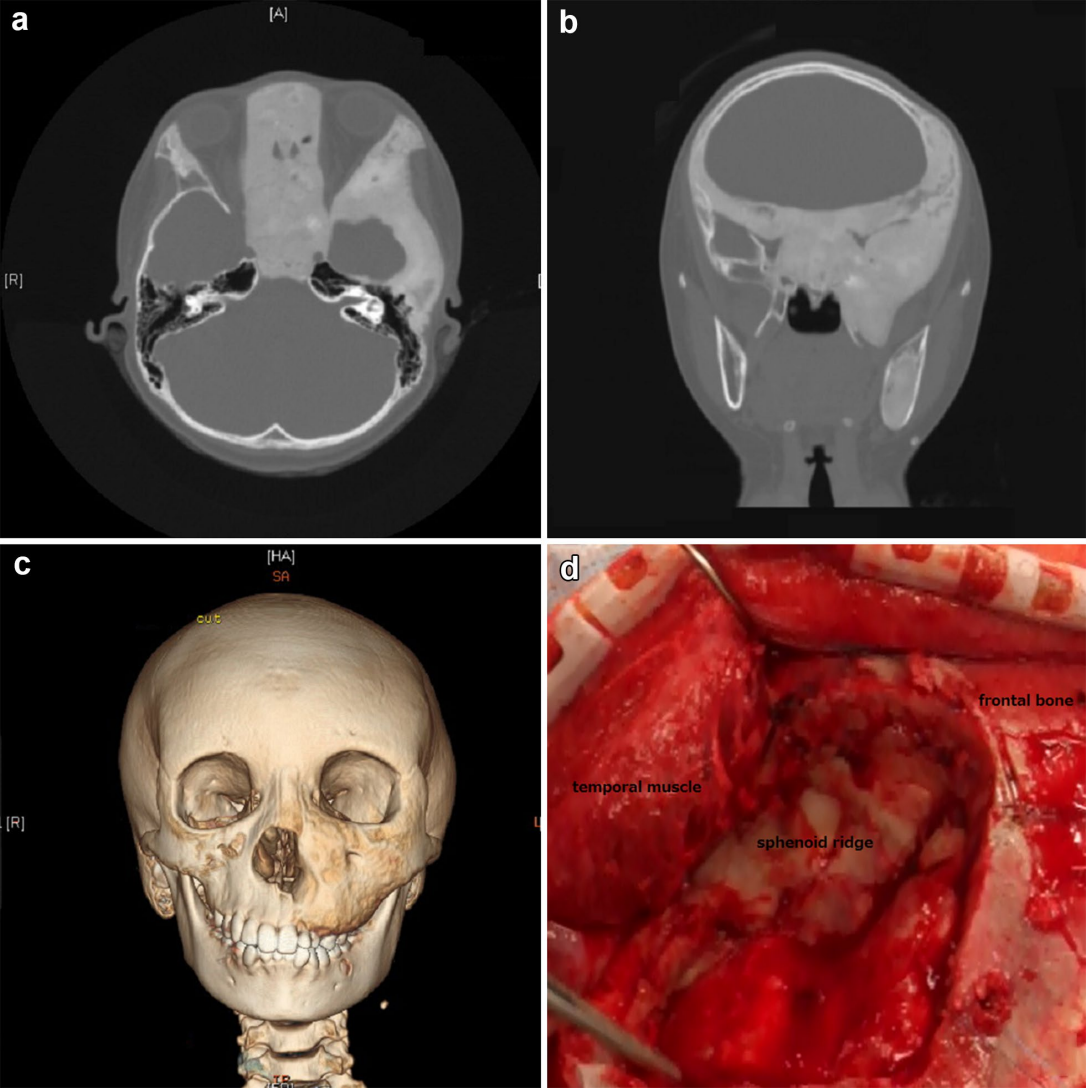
a High-resolution head axial CT shows extended bone hypertrophy of the *left* lesser wing of sphenoid bone and anterior clinoid process. b High-resolution head coronal CT shows narrowing of the *left* optic canal, which accounts for the visual acuity disturbance. c Bone 3 dimensional-CT shows prominence of the *left* facial bone and the marked difference relative to the *right* side. d The sphenoid bone is quite thick. It is difficult to identify the superior wall of the optic canal
